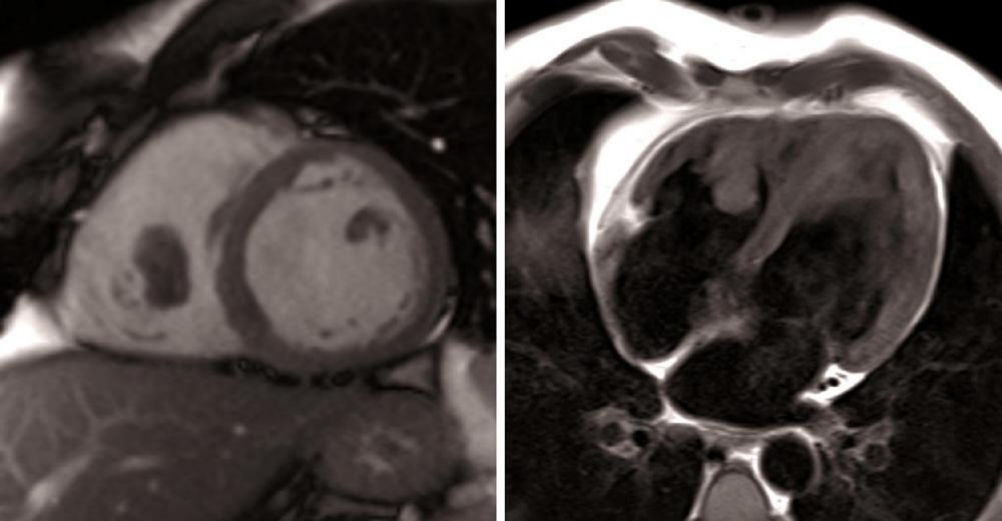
CMR showing thrombosis in the right ventricle. On the left: gradient echo sequences, short axis view. On the right side: T1-weighted sequence Double Inversion Recovery, four-chamber view.
